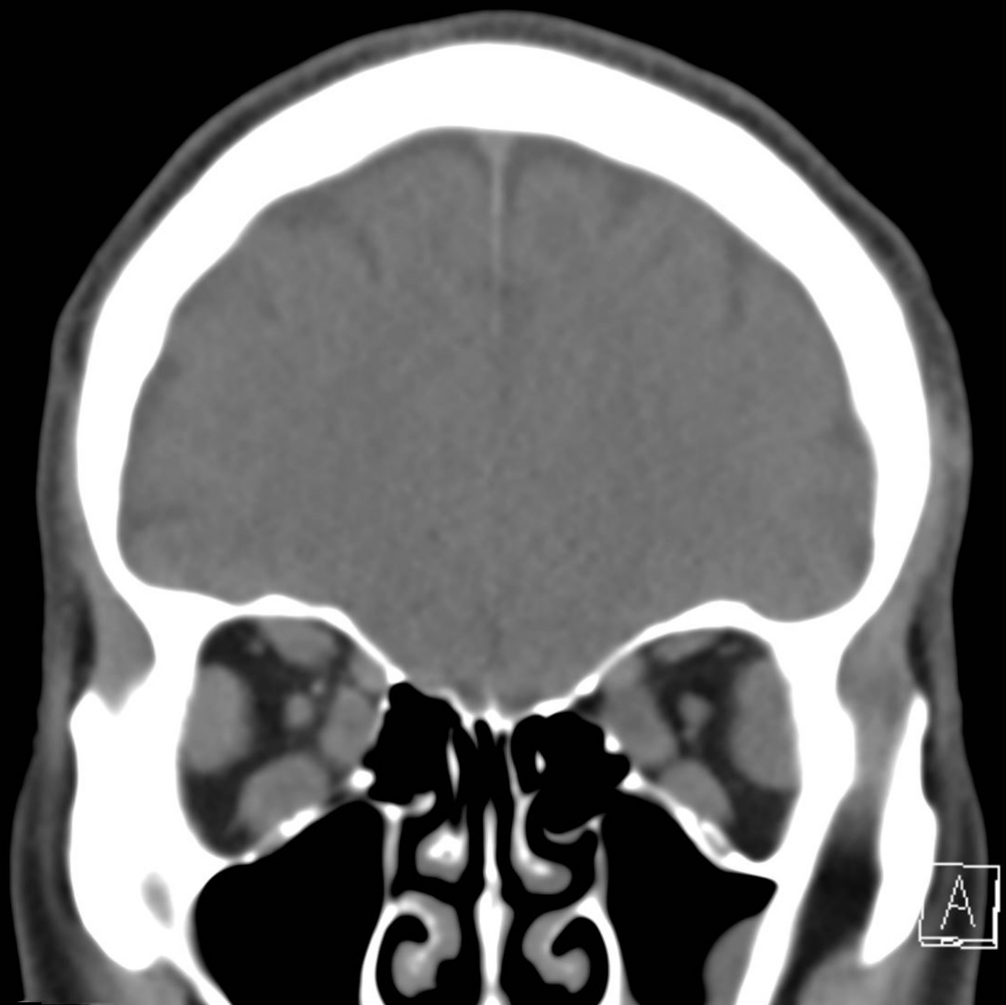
Coronal non-enhanced CT of the head demonstrates symmetric enlargement of the extraocular muscles in a 63-year-old male presenting with an acute episode of blurry vision and two months of headache and intermittent nausea.
